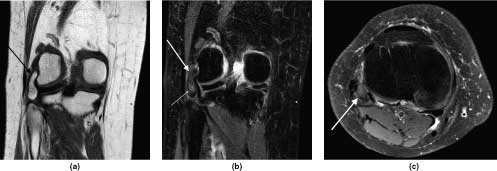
MRI images of the right knee. (a) *T*_1_ weighted coronal image showing a lipomatous mass in the lateral compartment of the knee joint, located between the lateral collateral ligament and the popliteus tendon (black arrow). (b,c) Fat suppressed *T*_2_ weighted coronal and axial image showing the lipomatous mass (large white arrows) with subtle signs of inflammation (small white arrow in b).
